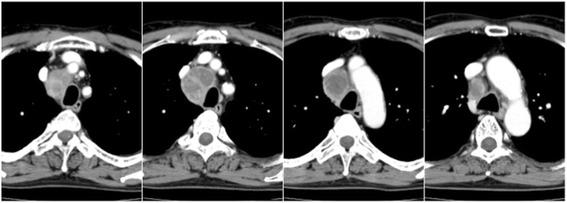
Chest CT before EBUS-TBNA showing swelling of the lower paratracheal lymph nodes. The lymph nodes are mostly necrotic
